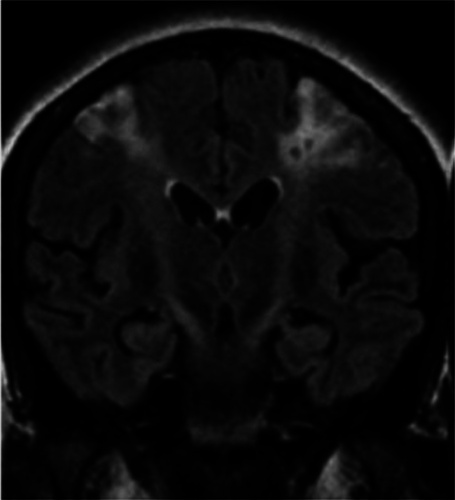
Plain MRI of the brain showing hyperintensity in bilateral watershed infarcts between bilateral anterior cerebral artery and middle cerebral artery territories.
